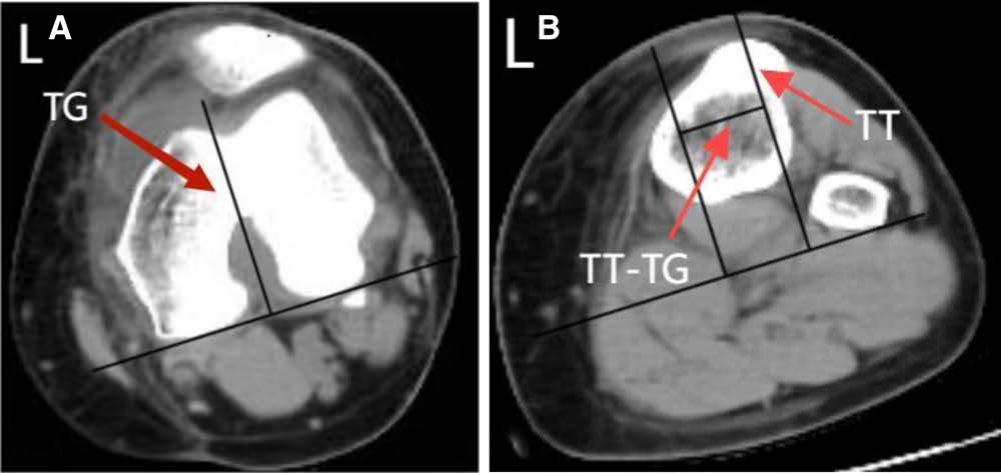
(A and B) Computed tomography measurement of the tibial tubercle-trochlear groove distance (TT-TG distance) according to ISAKOS standards. (A) Axial slice at the level of the deepest point of the femoral trochlear groove (point G). The PFCL is drawn. A perpendicular line to the PFCL passes through Point G. (B) Axial slice at the level of the peak of the tibial tubercle (Point T). The PFCL from (A) is maintained as the common reference axis. A perpendicular line to the PFCL passes through Point T. The TT-TG distance (31 mm) is measured as the distance between the 2 perpendicular lines on superimposed images aligned using the PFCL. Measurement performed using the PACS system electronic distance tool. PACS = picture archiving and communication system, PFCL = posterior femoral condylar line.
